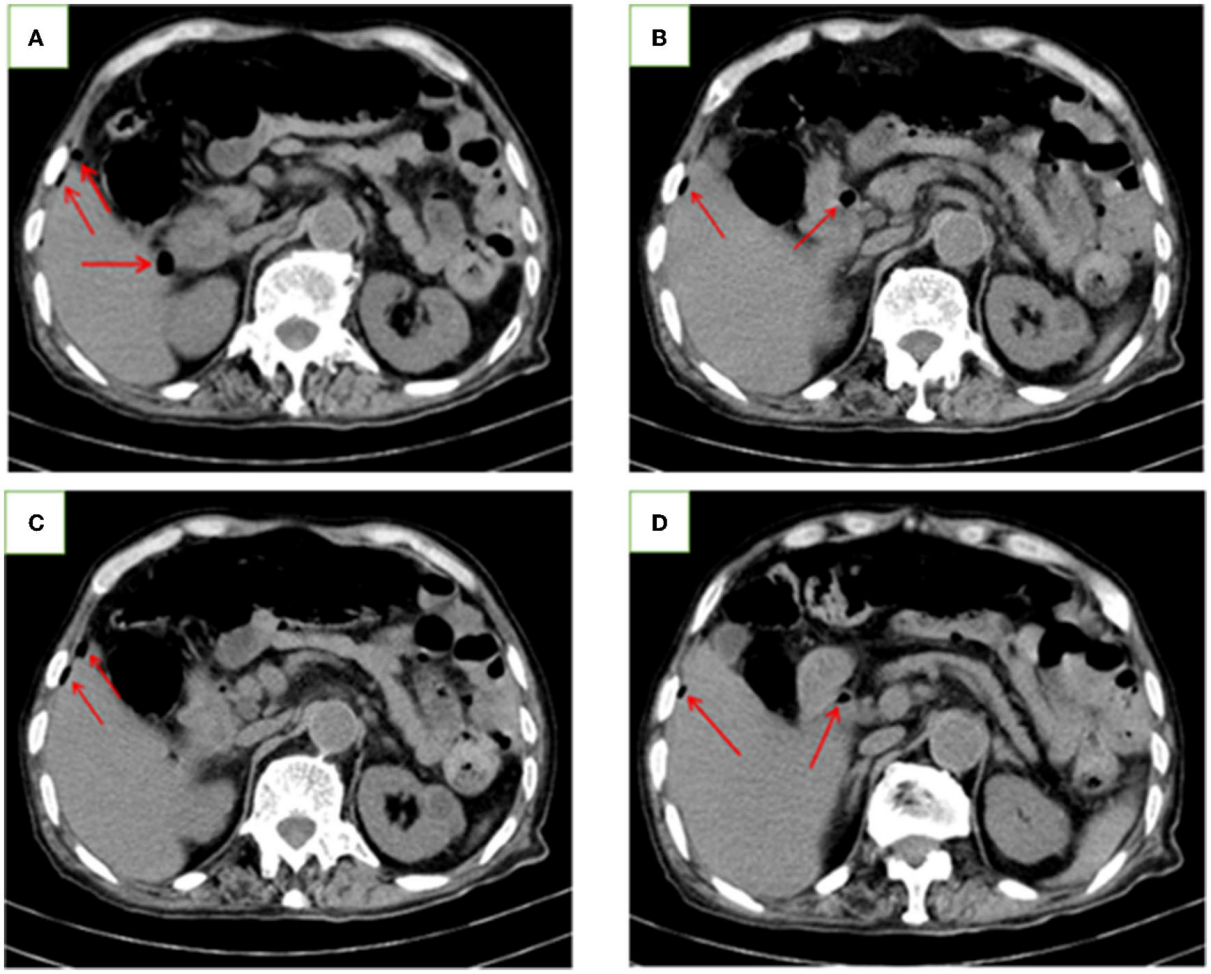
Abdominal CT images of the patient. (A–D) Abdominal CT scan showing the free gas (red arrow) in the abdominal cavity.
